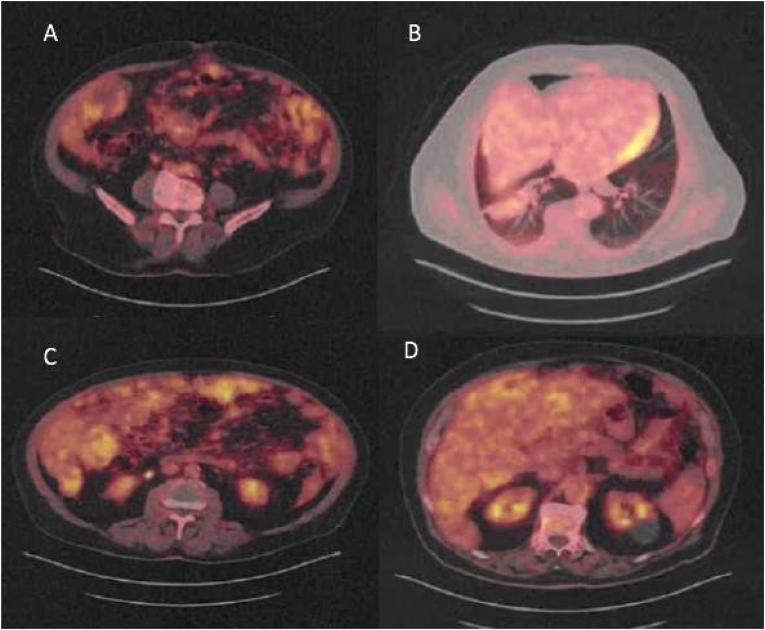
Positron emission tomography-computed tomography (PET/CT) finding. The nodular mass lesion shows intense FDG uptake in segment VII (B, D). Moderate to intense FDG uptake on large peripheral peritoneal structures (A, C).
